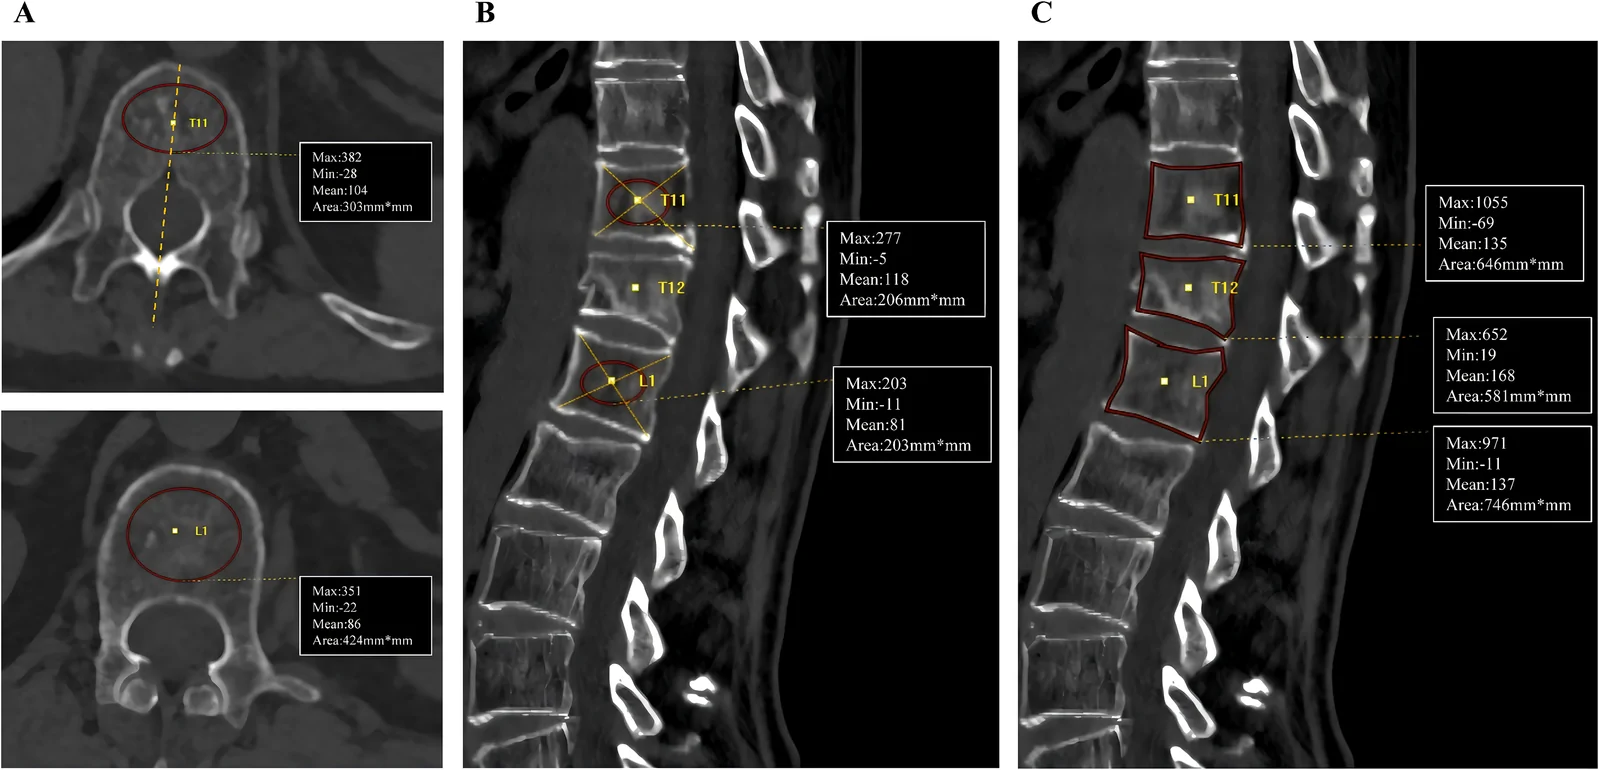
Measurement of the CT Hu value and SACR-FVB. (A) Axial CT Hu of the adjacent vertebral body. (B) Sagittal CT Hu of the adjacent vertebral body. (C) The FSCSA and the adjacent non-fractured vertebral bodies (NSCSA above and below). Fractured vertebral body CT Hu = (axial + sagittal CT Hu of superior vertebra + axial + sagittal CT Hu of inferior vertebra) / 4. The mean NSCSA was calculated as follows: NSCSA_mean_ = (NSCSA_above_ + NSCSA_below_) / 2. SACR-FVB was then calculated using the following formula: SACR-FVB = (NSCSA_mean_ – FSCSA) / NSCSA_mean_.
